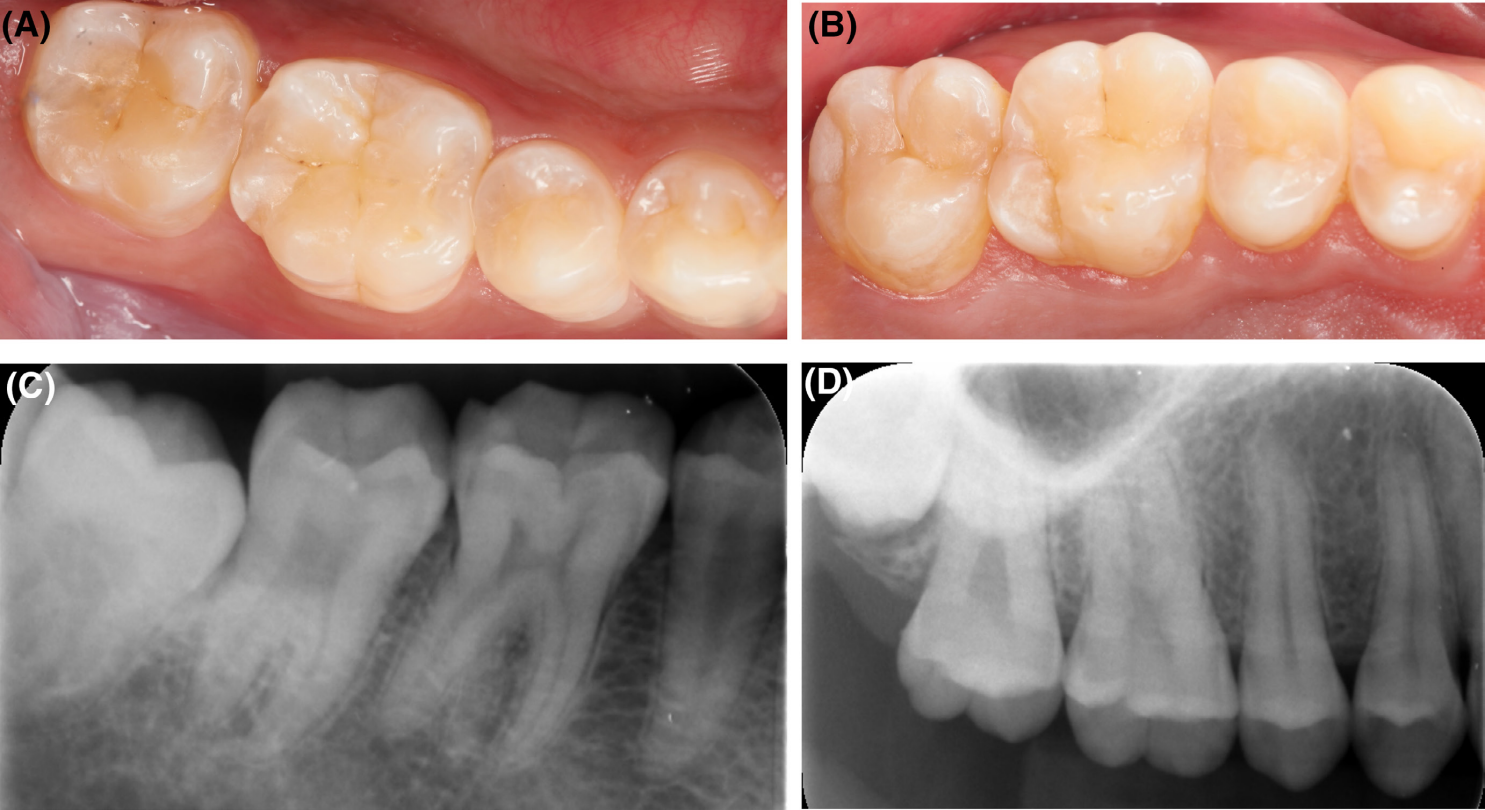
(A) Clinical presentation of the mandibular right molar region. All teeth were healthy and caries‐free, with age‐appropriate signs of attrition and without previous restorations. (B) Clinical presentation of the maxillary right molar region. The #16 tooth had a steep cusp with a crack line on the distal–lingual cusp. (C, D) The periapical radiograph of mandibular (C) and maxillary (D) right molar region. The X‐ray films revealed normal width of the periodontal ligament and without periapical lesion.
